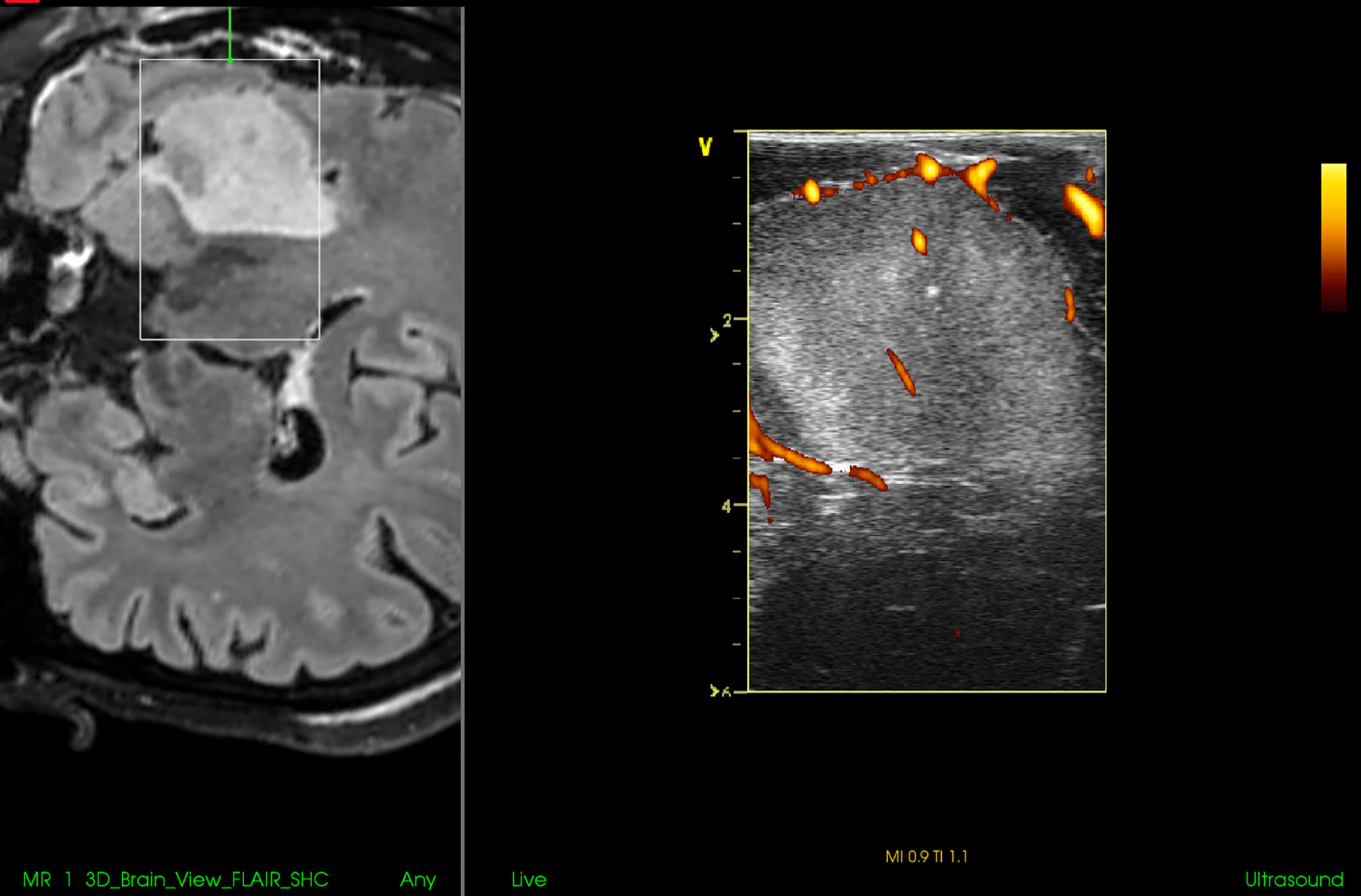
Right-sided insular grade II astrocytoma. Visualization of the tumor before the resection using 12 MHz linear IOUS probe co-registrated with preoperative navigation 3D FLAIR MRI sequence. Note co-registration with navigation MRI facilitates anatomical orientation. 3D, three-dimensional; FLAIR, fluid attenuated inversion recovery; MRI, magnetic resonance imaging; IOUS, intraoperative ultrasound.
